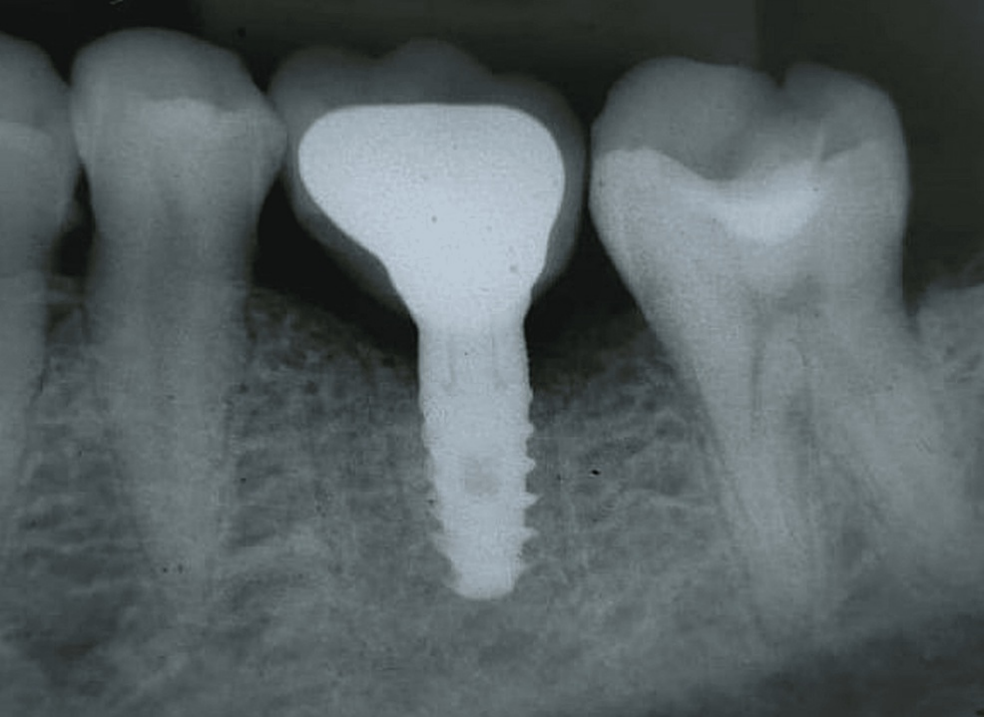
Radiograph of the implant placed after prosthesis
